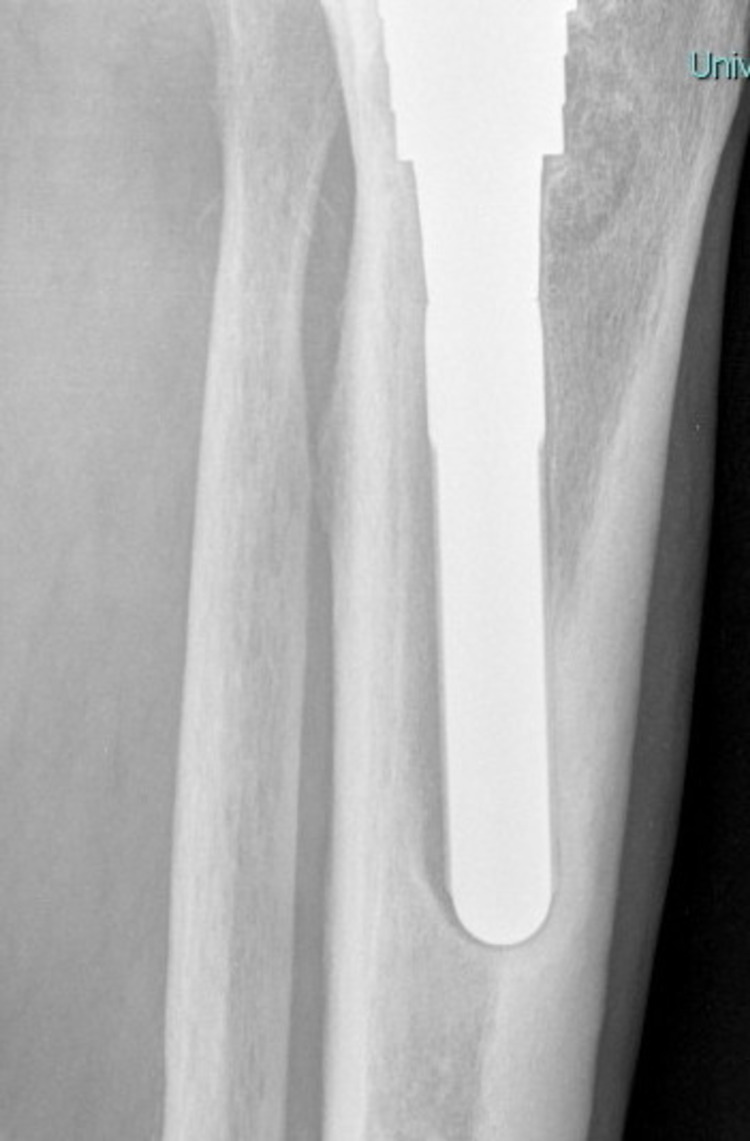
A 72-month follow-up X-ray lateral stem tip view. The tibial component has loosened and migrated into varus.
